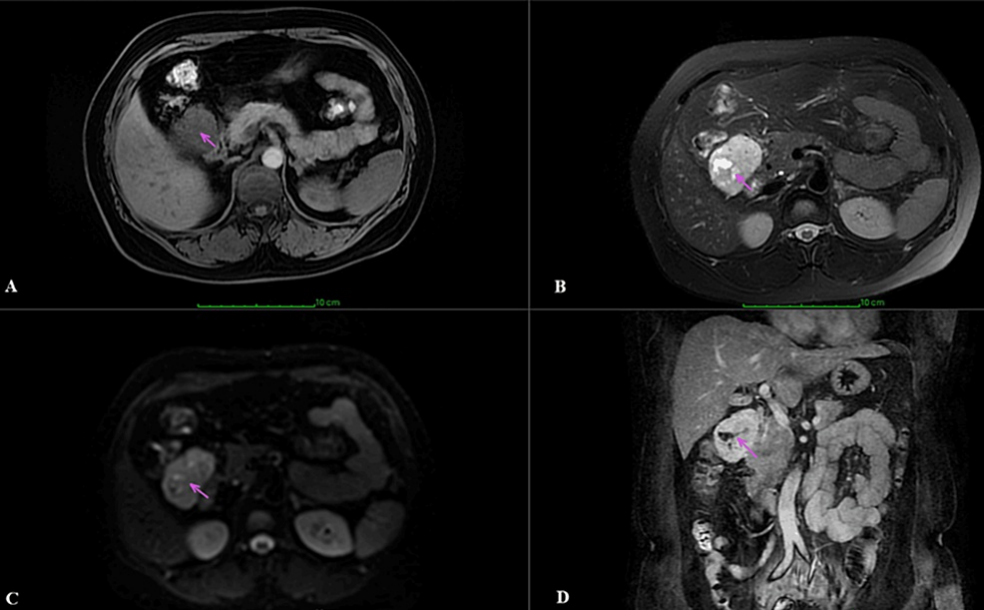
The imaging features of this mass on magnetic resonance imaging. (A) Low signal intensity lesion on T1-weighted image. (B) High signal intensity lesions on T2-weighted images. (C) High signal intensity lesion on DWI image. (D) Coronal T1-weighted enhancement scan with marked inhomogeneous enhancement.
